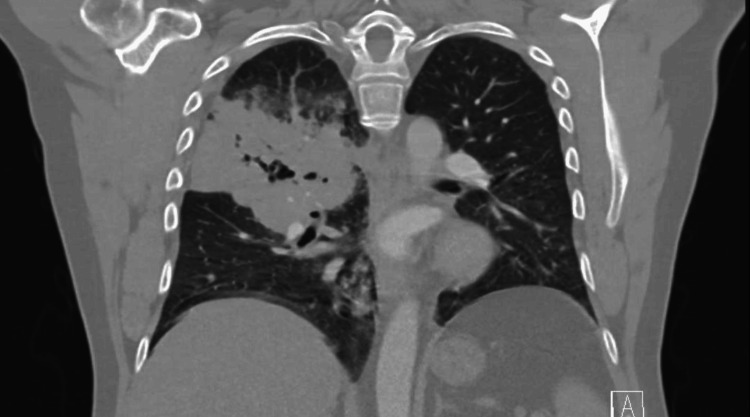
Coronal CTA chest image demonstrating dense consolidation in the right upper and middle lobes of the lung. CTA, computed tomography angiography
